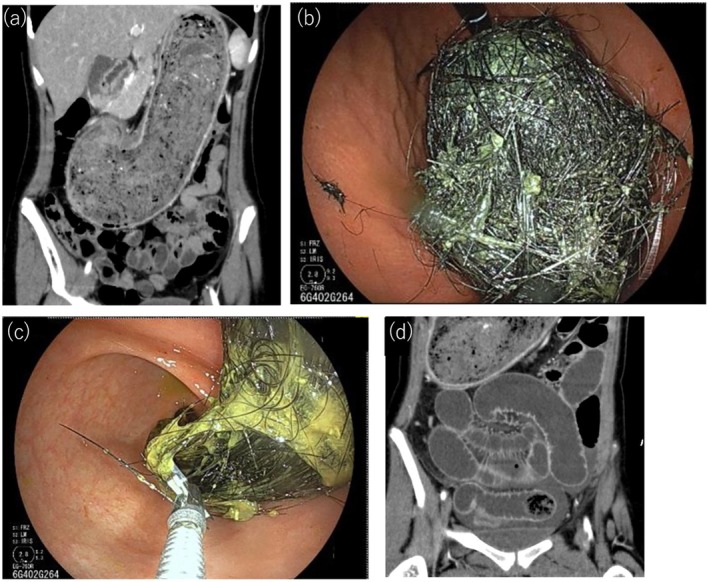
(a) Contrast‐enhanced abdominal CT showing a large heterogeneous intragastric mass containing air and high‐density components, consistent with a trichobezoar. (b) Upper gastrointestinal endoscopy demonstrating a large hair mass occupying the gastric cavity and extending beyond the pylorus. (c) Endoscopic fragmentation of the trichobezoar using a bipolar snare and electrocautery. (d) Repeat contrast‐enhanced CT showing small bowel obstruction due to migration of trichobezoar fragments to the ileum.
